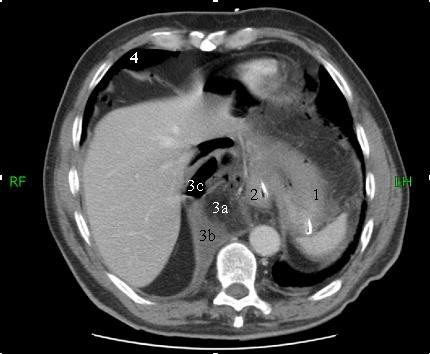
CT-scan. Axial 5 mm CT-slice after i.v. contrast admission. This slice shows an intra-abdominal situated stomach with nasogastric tube (1); esophagus with NGT (2); right-sided para-esophageal hernia with intraperitoneal fat (a), free-fluid (b) en free-air (c) (3); intra-peritoneal free-air (4).
